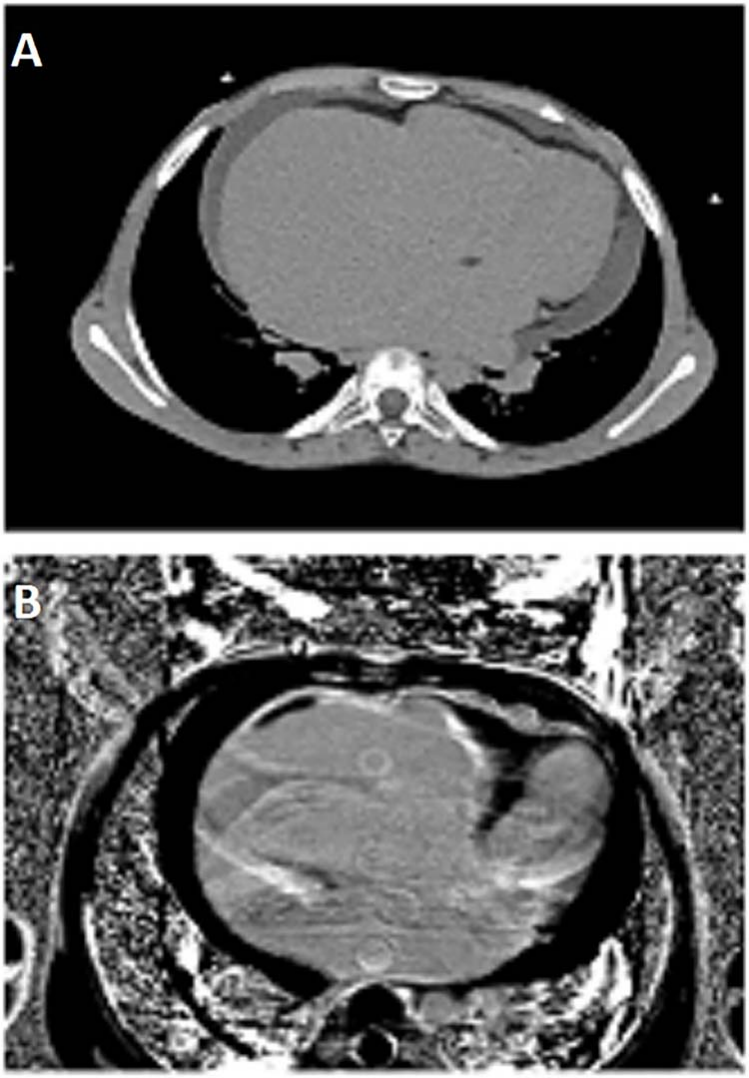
(A) Cardiac CT-scan revealing severe dilation of the right atrium associated with thrombosis in right atrium and ventricle. (B) CMR: 4-chambers view LGE sequence showing right ventricular endomyocardial fibrosis, with enhancement alongside thrombosis consistent with typical ‘three-layered’ pattern.
